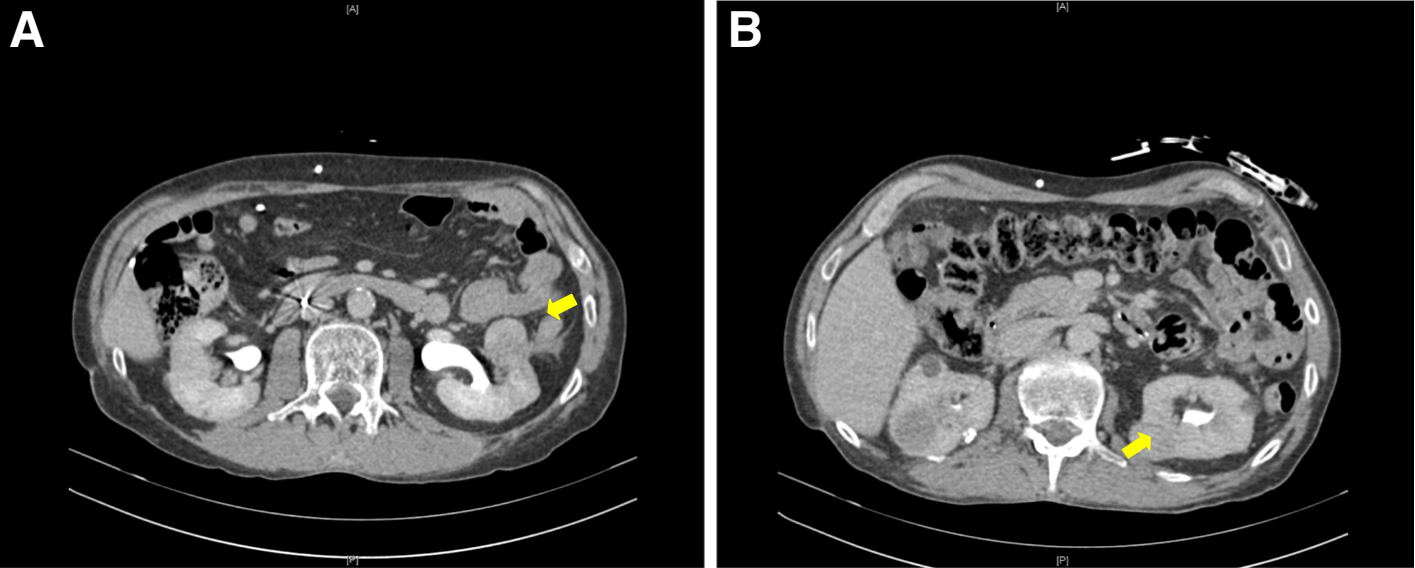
(A, B) Computed tomography scan shows complete resolution of the fistula after treatment, resolution of pericolic fat stranding, and disappearance of gas bubbles
